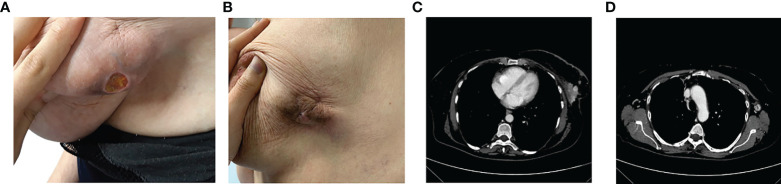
After the II cycle, the ulcerated was decreased (A), up to the III cycle when there was the cutaneous resolution of the ulcerated mass (B), with a partial response at the CT scan (C) and a complete response of pathological lymph nodes (D).
